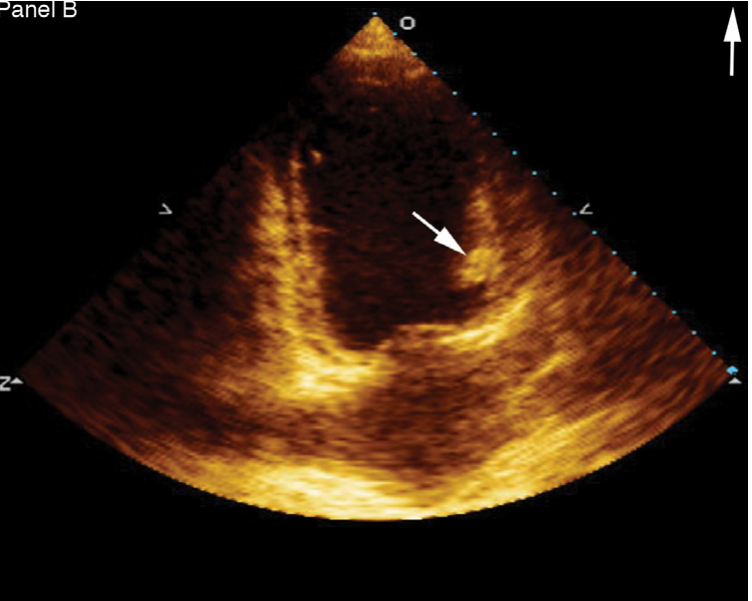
Transthoracic echocardiography showing left ventricular thrombus (arrow).
